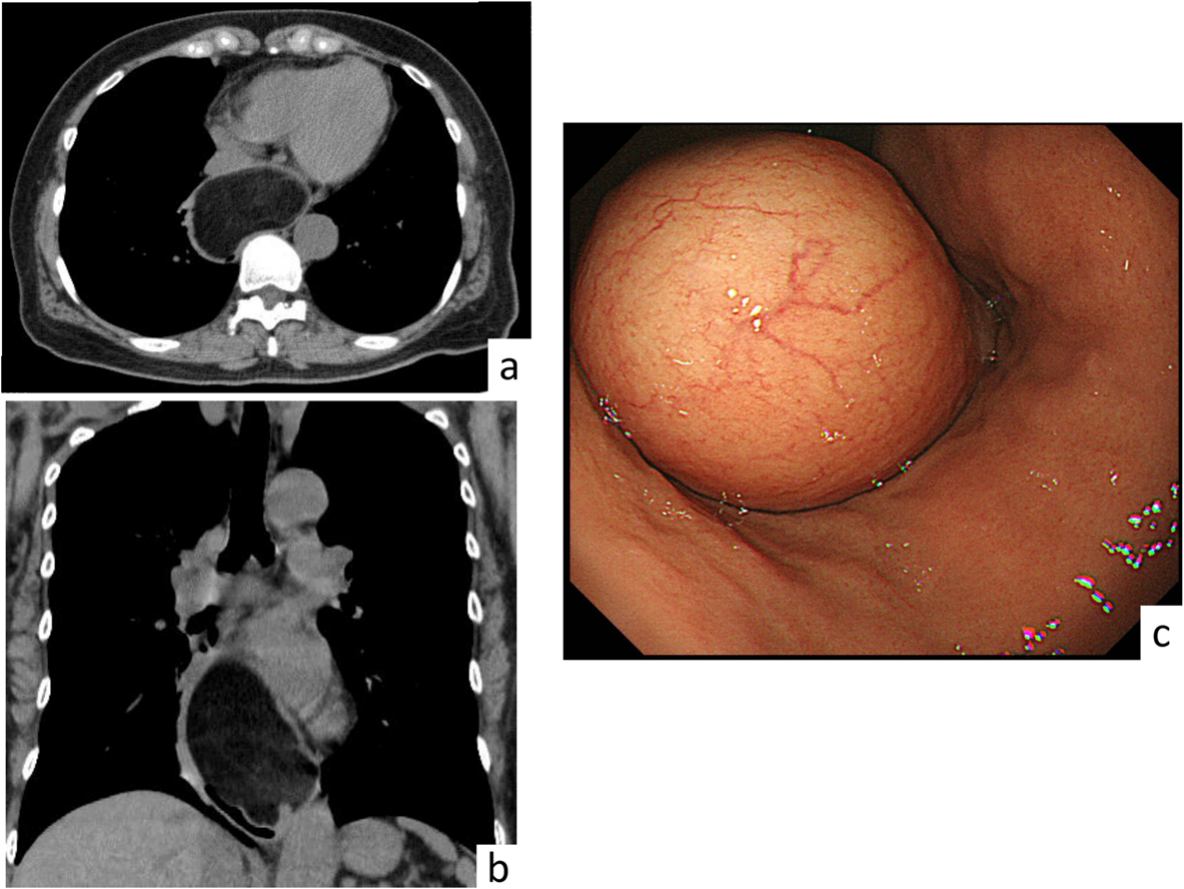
Preoperative imaging. (a horizontal, b coronal) CT scan of the chest revealed a 10 × 7 cm homogenous mass in the middle and lower esophagus. c Upper endoscopy revealed a submucosal tumor with normal mucosa arising from the left esophageal wall
